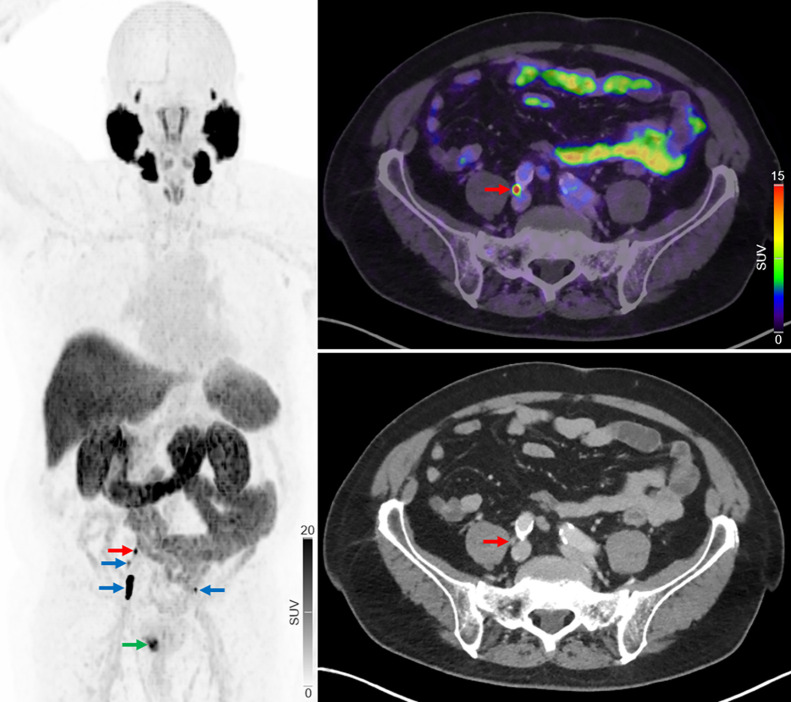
Primary staging of patient with high-risk PC. Initial ^18^F-NaF PET/CT showed no regional or distant metastases. Patient later underwent radical prostatectomy with extended pelvic lymph node dissection. In blinded project review, ^18^F-PSMA PET/CT revealed multiple intrapelvic lymph node metastases (blue arrows) and 1 lymph node metastasis near bifurcation of right common iliac artery (red arrows). Primary prostate lesion is also visible (green arrow). Three months postoperatively, patient’s PSA remained elevated (0.67 ng/mL), and bicalutamide therapy was started.
